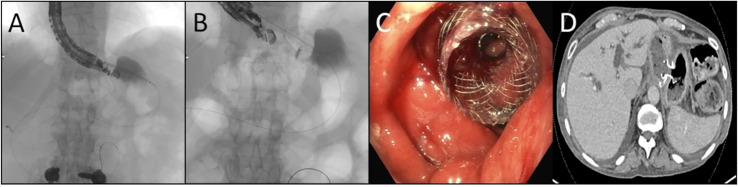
Endoscopic Reversal of Roux-en-Y (A) The remnant stomach is localized by endosonography and subsequently accessed by passing a catheter preloaded with a stent across the pouch or Roux as well as the wall of the remnant using electrocautery (B) Using combination of ultrasonography, fluoroscopy, and endoscopy the stent is deployed with each bell expanding in the desire lumen (C) Following deployment the covered lumen apposing metal stent is found well seated with the remnant seen across the saddle which may be subsequently dilated (D) Axial computed tomographic image demonstrating the stent positioned across the pouch and remnant.
